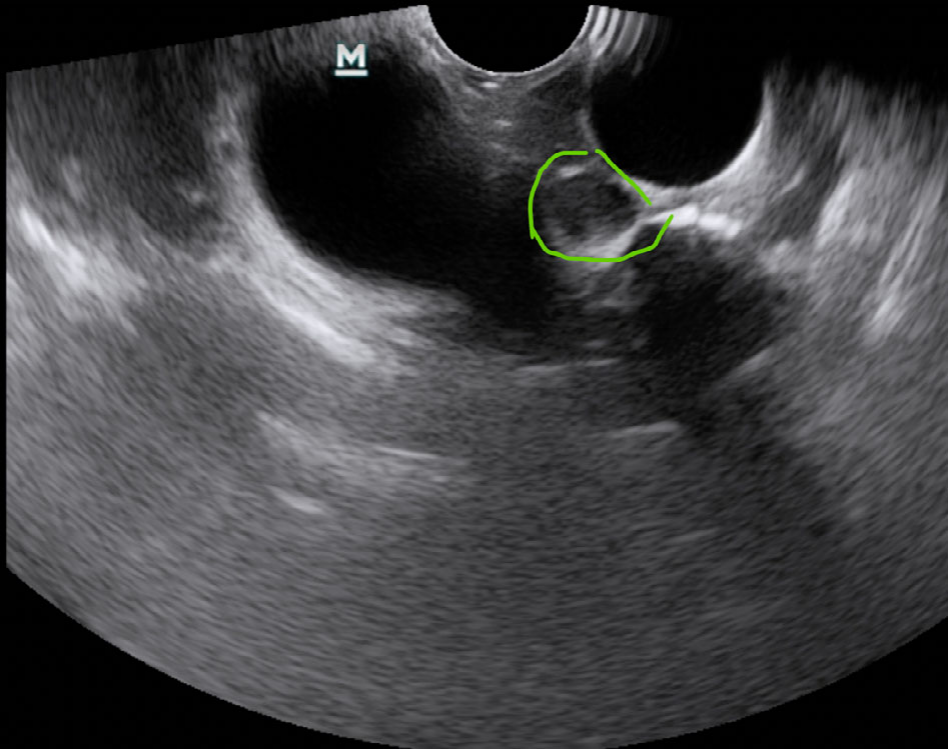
Pathological nodal sonographic characteristics.
